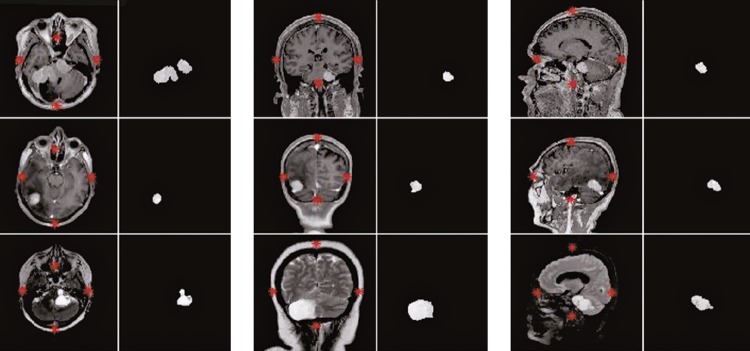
Final result of automatic segmentation in T1-, T2- and spoiled gradient echo contrasted T1-weighted magnetic resonance imaging
